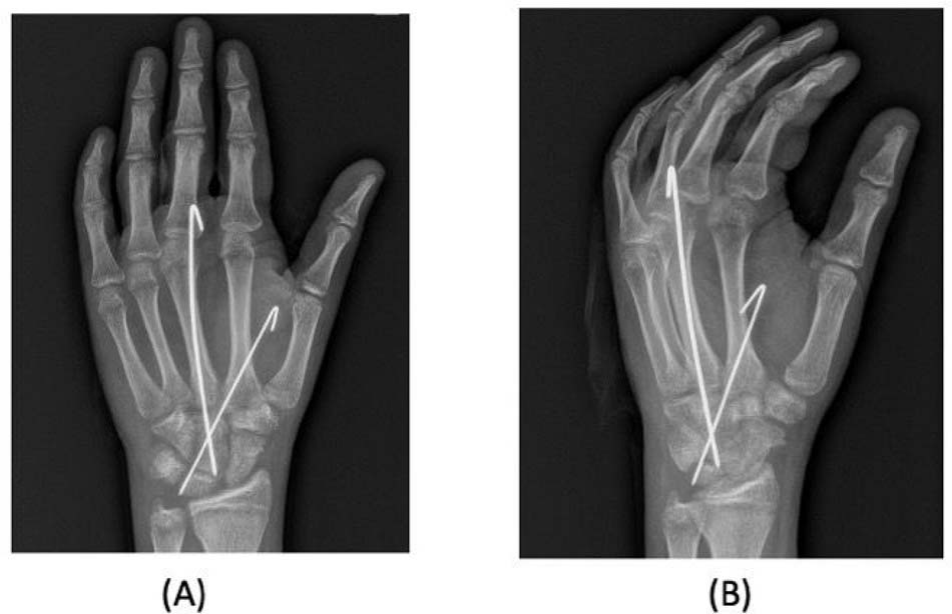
Posteroanterior and oblique radiographs taken intraoperatively after fixation, demonstrating proper alignment of hardware.
